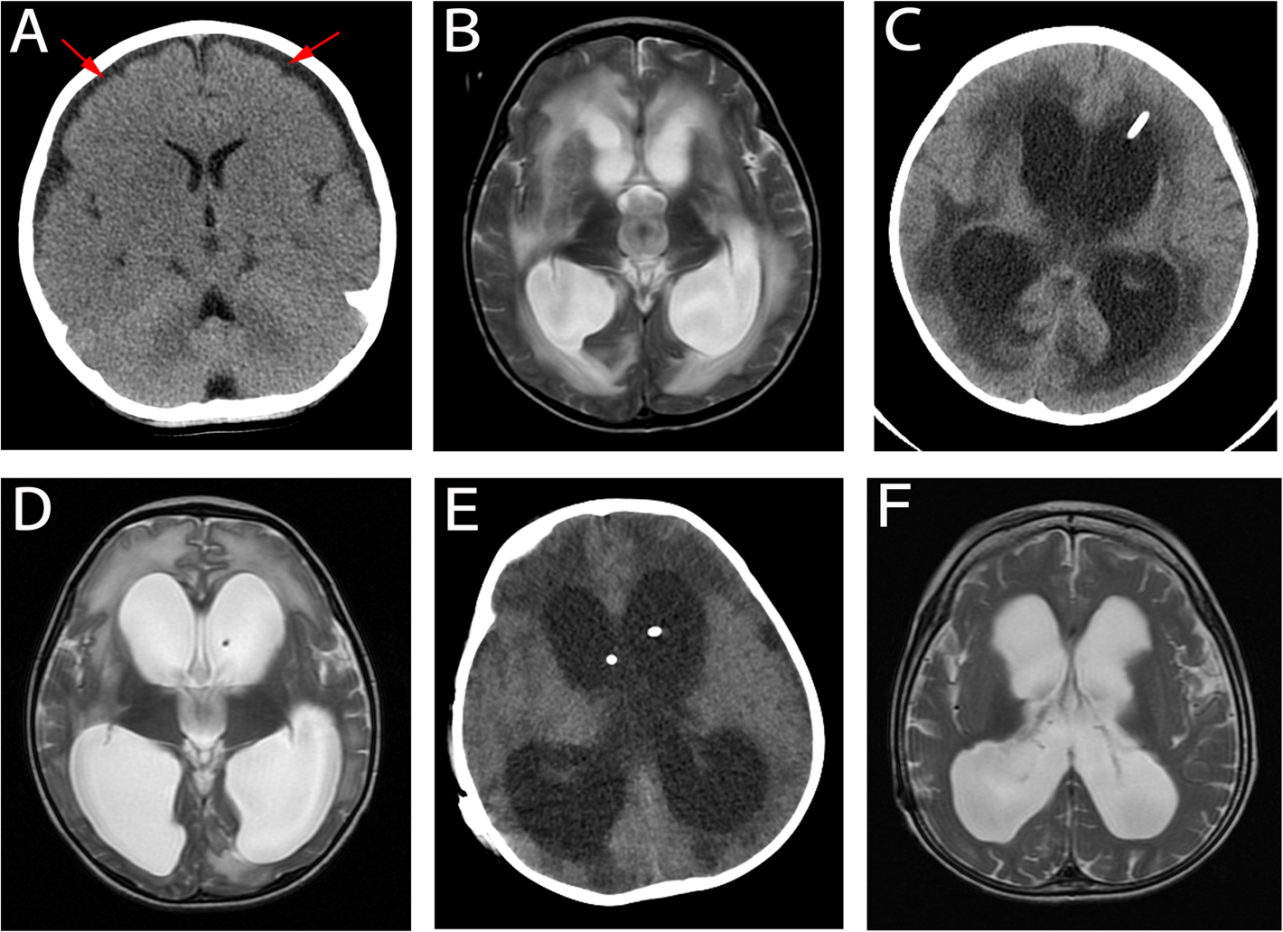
Serial CT and MRI images showing changes in the brain. a Day 19 of admission: Lateralcranial CT showing diffuse brain edema, hypodensity of the brain parenchyma, and subdural effusion in the bilateral frontal area (red arrow). b Day 25 of admission (one day before Ommaya reservoir implantation): Lateral T2-weighted MRI showing enlarged lateral ventricles and hydrops with peripheral white matter edema. c Day 27 of admission (one day after Ommaya reservoir implantation): Lateral cranial CT showing the drainage tube in the anterior horn of the left lateral ventricle. d Day 70 of admission (one day before ventriculoperitoneal shunt): Lateral T2-weighted MRI showing hydrocephalus in the ventricles was not obviously aggravated. e Day 72 of admission (one day after ventriculoperitoneal shunt): Lateral cranial CT showing the drainage tube in the lateral ventricle. e 20 months after discharge: Lateral T2-weighted MRI showing less hydrocephalus and improved interstitial cerebral edema
